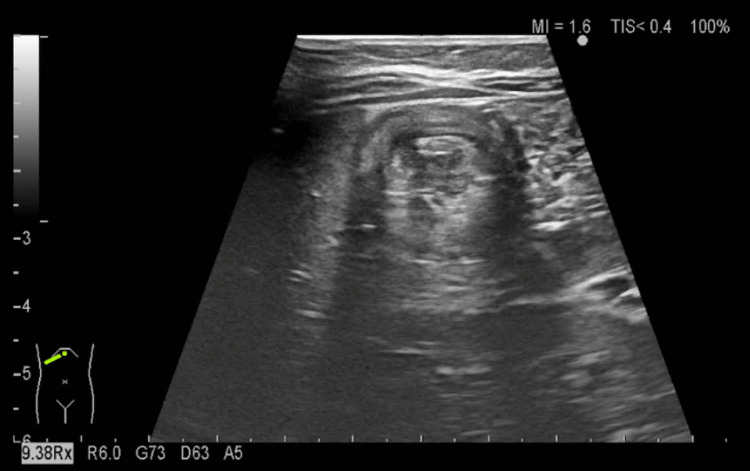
Abdominal ultrasonography Abdominal ultrasonography shows a “target sign” in the patient’s hepatic region. No echo patterns are observed in the surrounding areas
